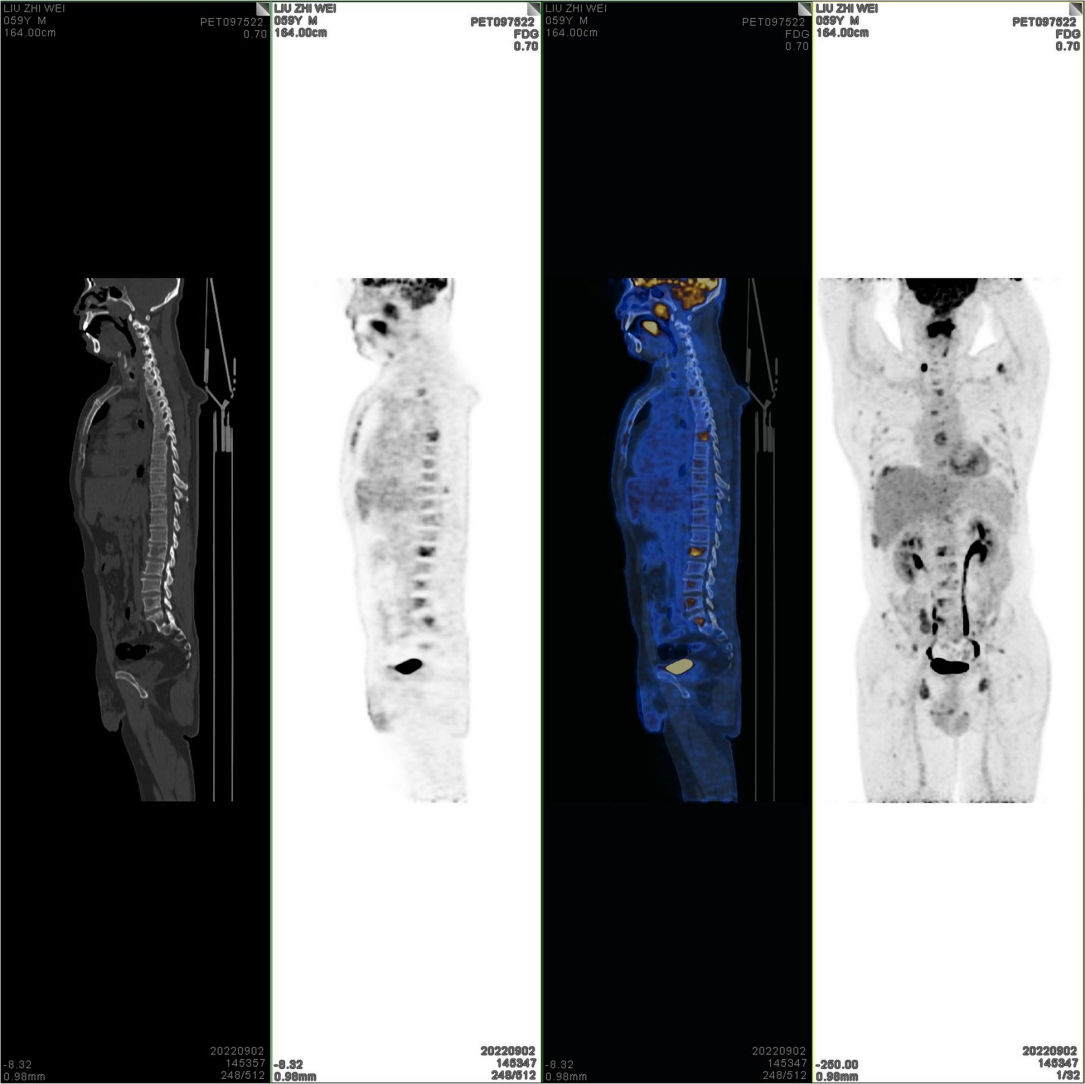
PET-CT scans revealed bone destructions in multiple vertebral bodies and appendix
